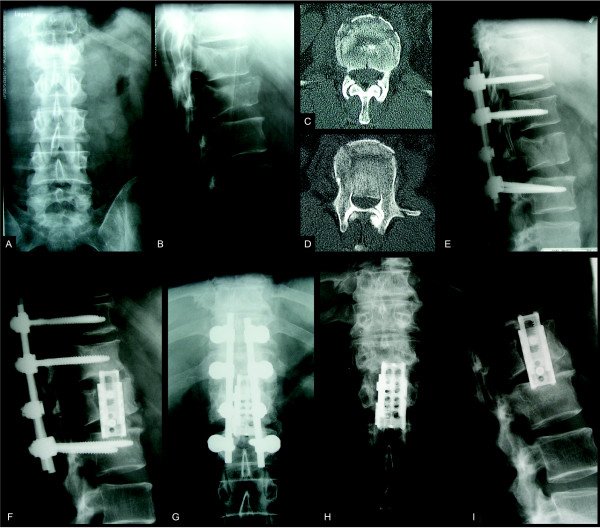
Conventional open reduction and instrumentation with secondary anterior surgery in a polytraumatized patient with compression fracture of T12 and complete burst fracture of L1. This case features a 39 year old male patient following a fall from height (ISS = 41). The patient was unconscious at the site of the injury and transferred after tracheal intubation to the trauma centre. Following primary survey and whole-body CT-Scan, severe traumatic brain injury with epidural hematoma, retroperitoneal bleeding with bilateral lung contusions and instable spine injuries from a complete burst fracture of L1 with substantial spinal canal compromise (type A3.3) and adjacent compression fracture of T12 (type A1.2) were revealed (images A-D). The patient was positioned prone and simultaneous surgery was performed for evacuation of epidural hematoma and stabilization of the spine. Posterior fusion using a conventional approach was performed to achieve optimized reduction of the posterior wall fragment and strongest stabilization using a cross-link and bone graft (image E). Following uneventful recovery from intracranial injuries, the patient was operated anterior using an expandable cage on day 10 post trauma (images F-G). Removal of the internal fixator after 14 months released cranial motion segment T11-T12 and showed sufficient bisegmental anterior fusion (images H-I). (Adopted from Heyde CE, Stahel PF, Ertel W. "Was gibt es Neues in der Unfallchirurgie" in: Meßmer, Jähne, Neuhaus: Was gibt es Neues in der Chirurgie? Ecomed Medizin 2005).
